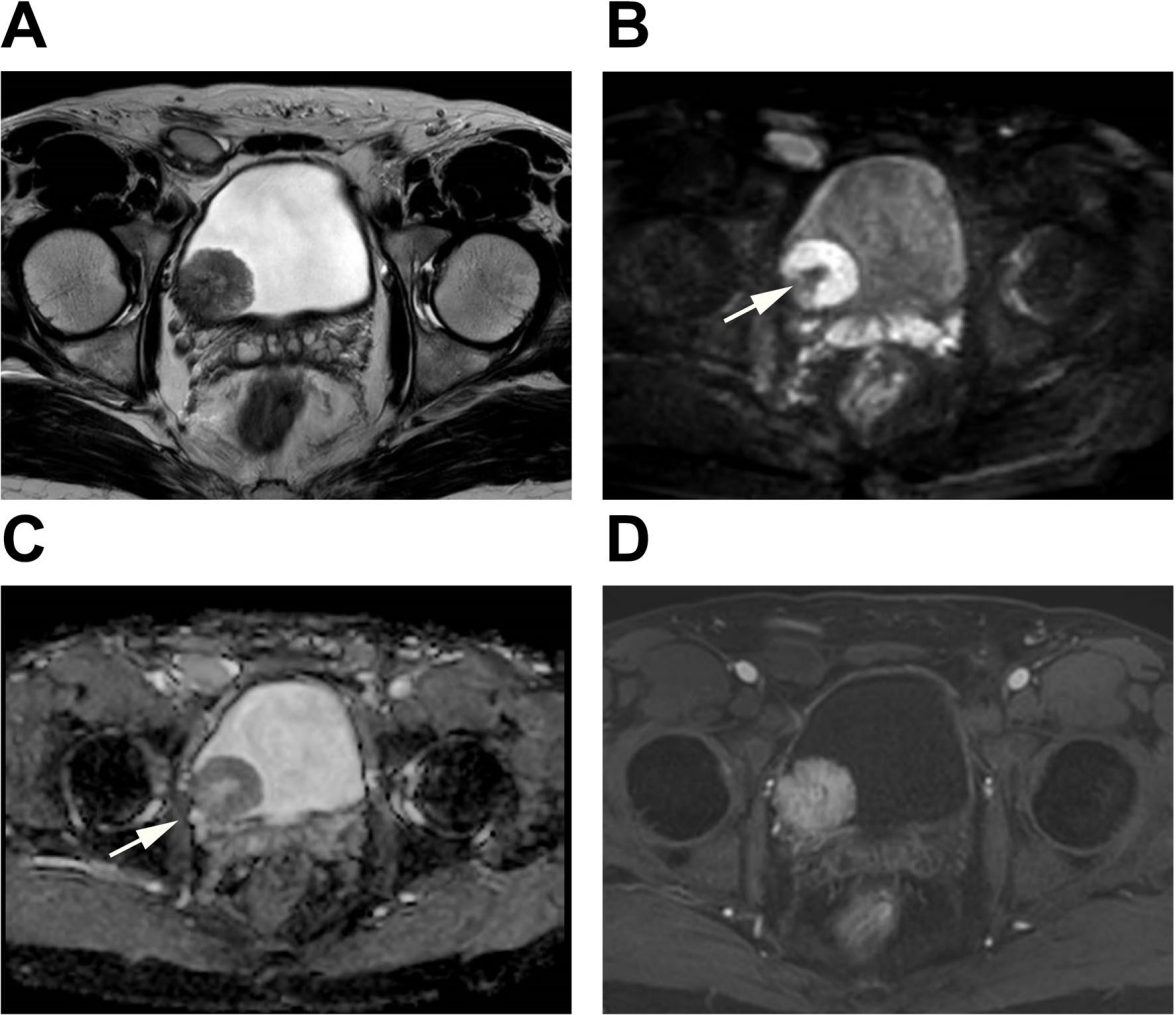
A case was evaluated using three evaluation methods. A patient over 40 years old underwent mp-MRI before primary TURBT. T2WI revealed a single tumor with a clearly defined high signal intensity stalk on the right posterior wall of the bladder. Tumors with a clear stalk were also identified on DWI and the ADC map (Arrow). All readers assigned a T2WI score of 2 and a DWI score of 2, resulting in both bp-DWI and bp-T2WI VI-RADS scores being 2. DCE did not show early enhancement of the muscularis propria. Therefore, all the readers assigned a DCE score of 2, resulting in a VI-RADS score of 2 for mp-MRI. T stage after TURBT was T1-high grade with squamous differentiation. A: T2WI; B: DWI; C: ADC; D: DCE
